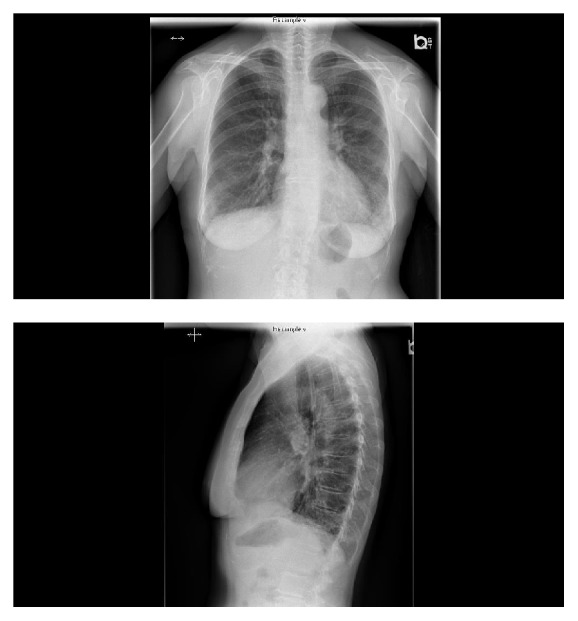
Posteroanterior and lateral chest X-ray revealing bilateral reticular infiltrates seen more significantly in the lower lung zones.
